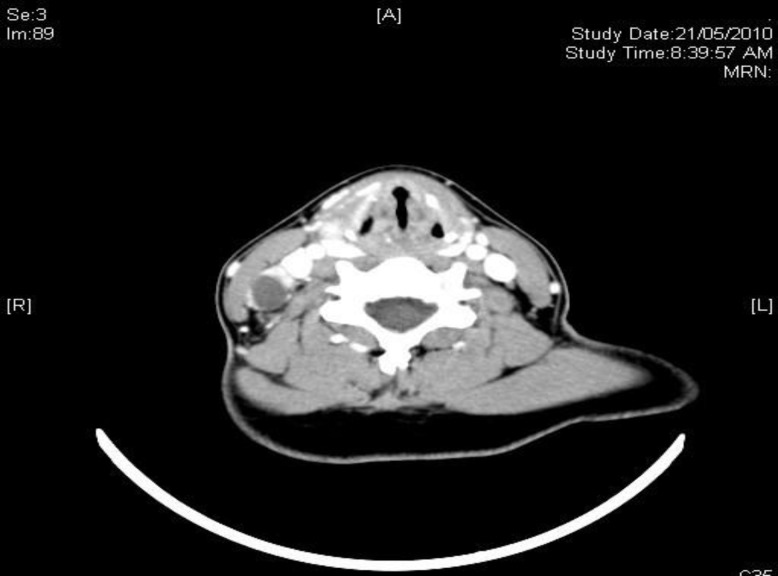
Axial CT scan of the neck demonstrating multiple cervical lymph nodes, with normal-looking thyroid gland.
